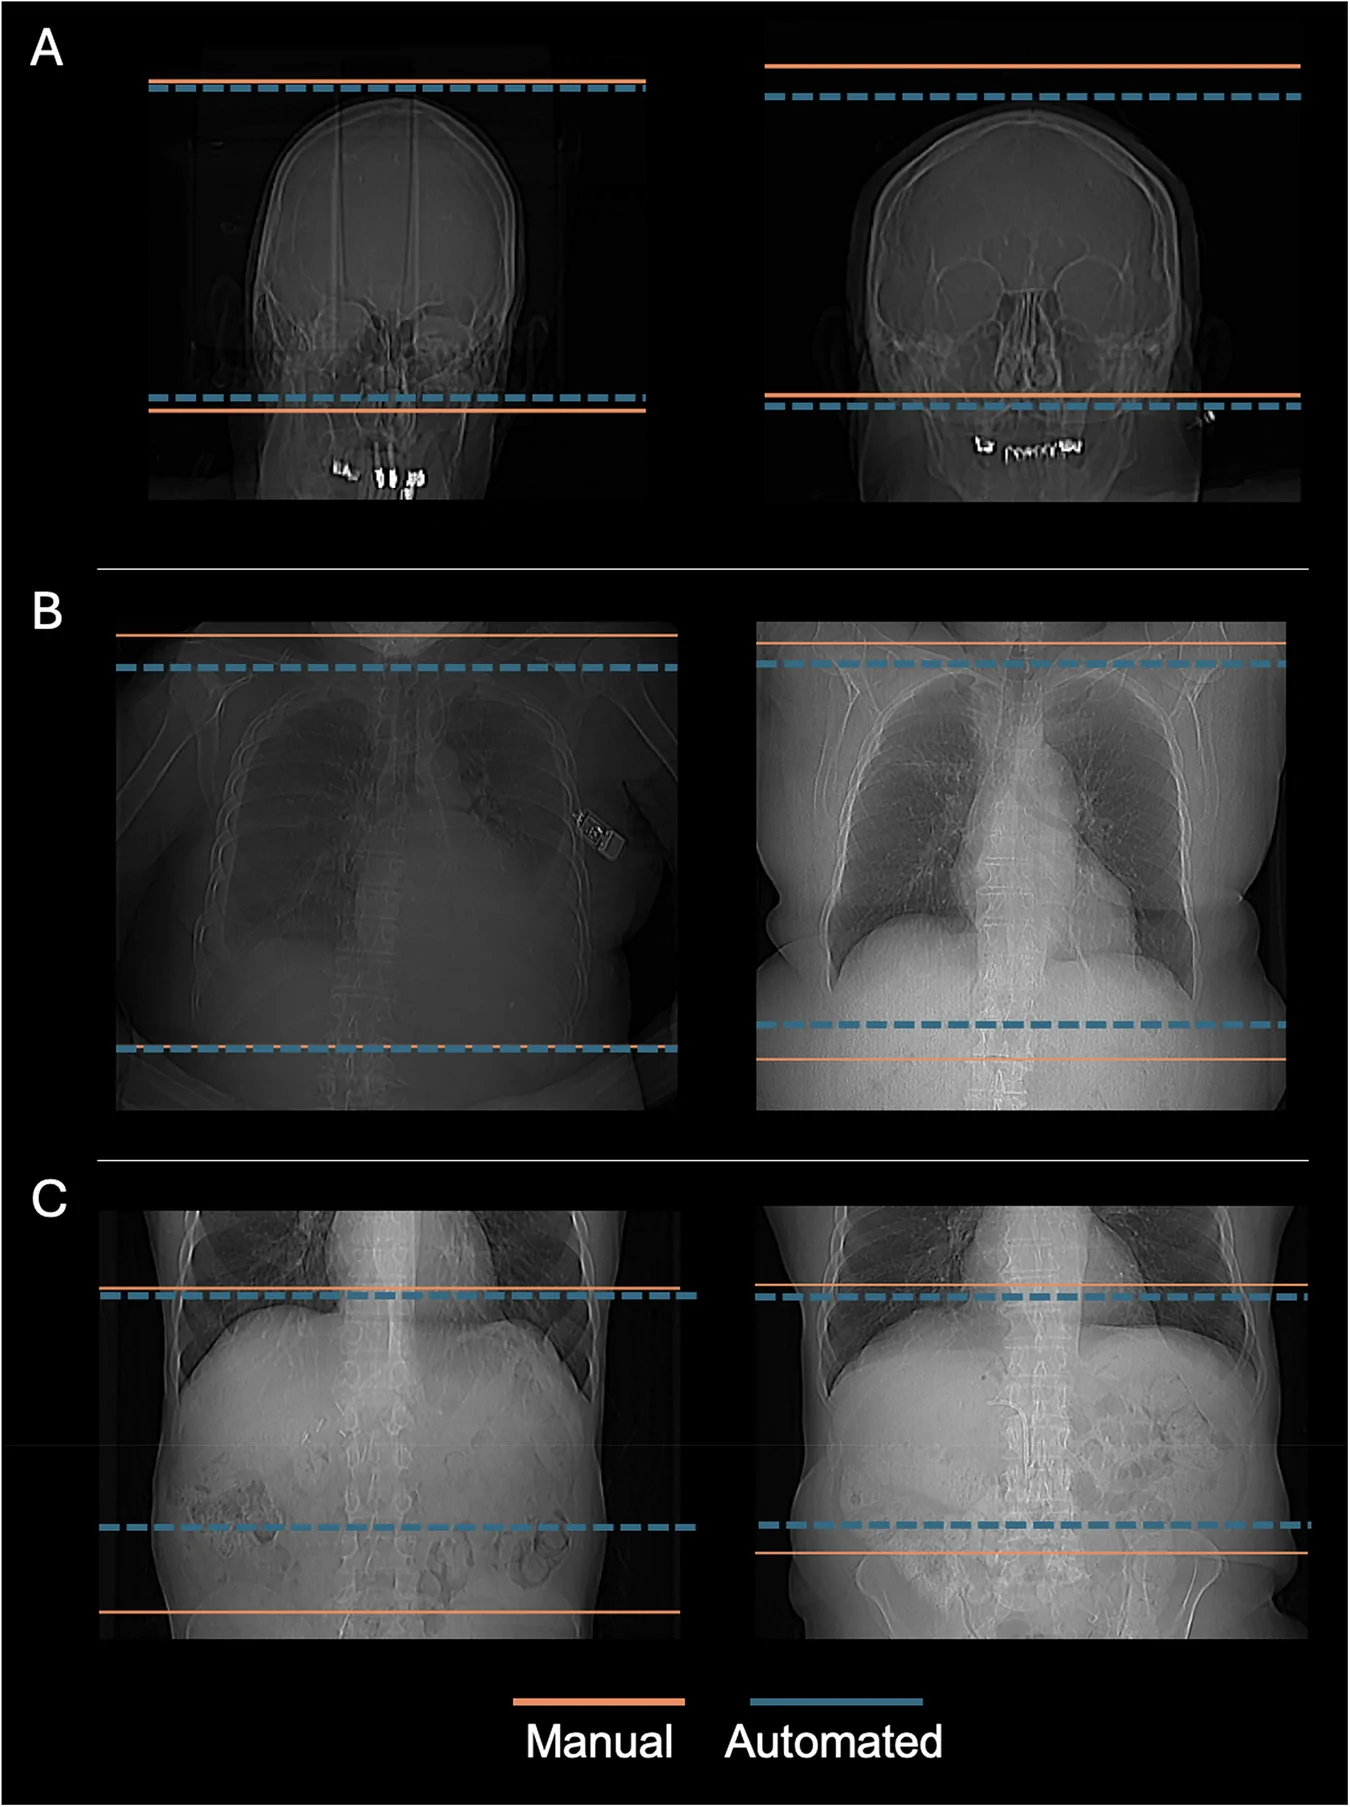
Representative scout views comparing manual and segmentation-derived scan-range planning for cranial (A), chest (B) and upper abdominal (C) CT examinations
